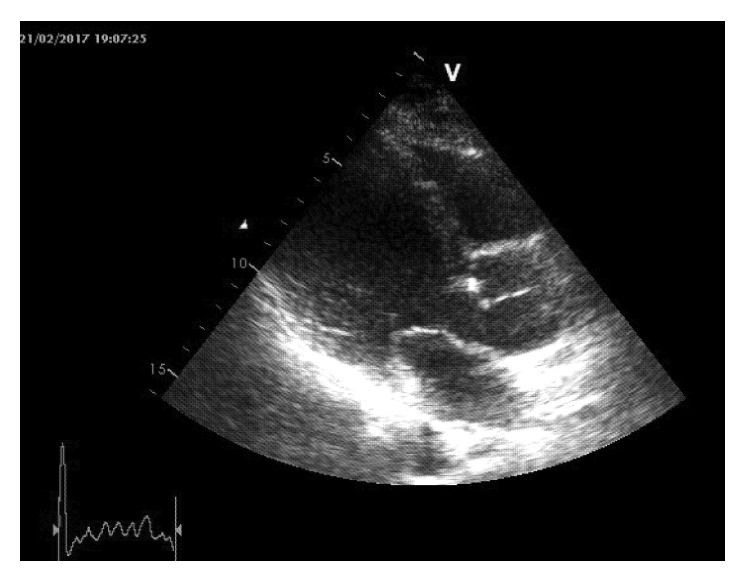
Transthoracic ultrasound showing a degenerated bicuspid aortic valve, combined with left ventricle dilation. A small mass on the left aortic cusp is distinguished as septic vegetation, due to *L. lactis*.
